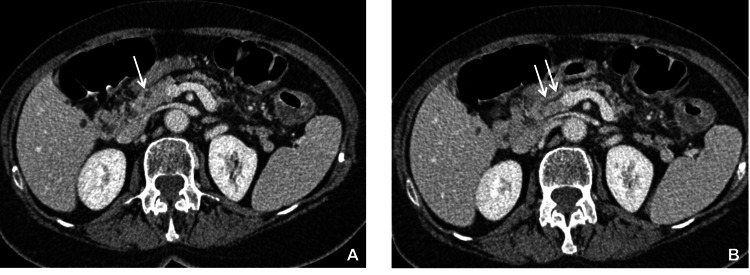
Abdominal contrast medium computed tomography of 2011 (A) Arrow indicates a minute cyst in the head of the pancreas, suspected for type1-IPMN, that is, in relation with the Wirsung duct. (B) The two arrows indicate a slightly dilated Wirsung duct IPMN: intraductal papillary mucinous neoplasm
